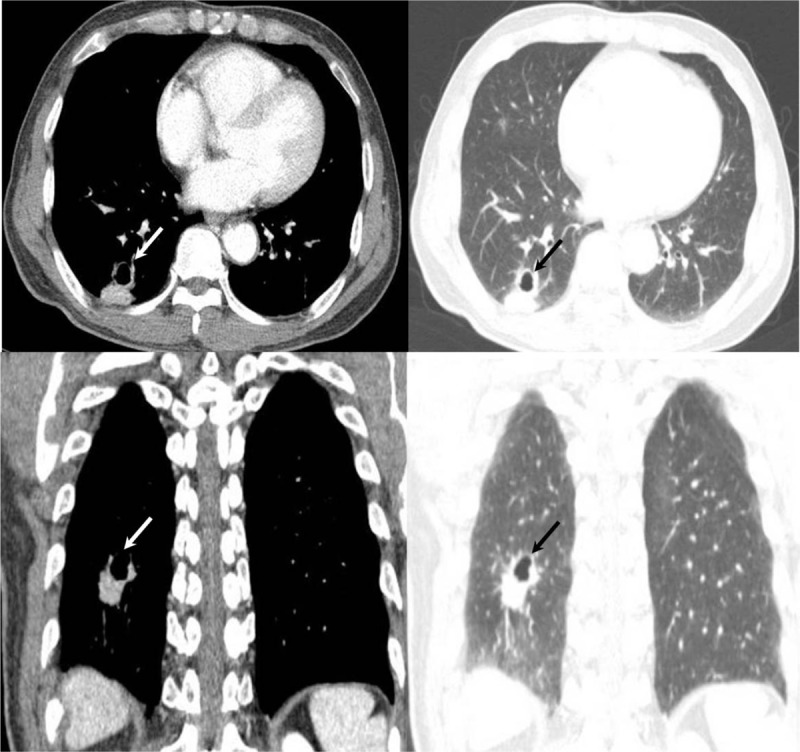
(A–D) Initial chest CT on health screening revealed a 3.2 cm cavitary lesion in the right lower lobe that consisted of an area of consolidation and cavity (white arrow indicated cavitary mass in RLL on mediastinal window setting, black arrow indicated cavitary mass in RLL on lung window setting).
